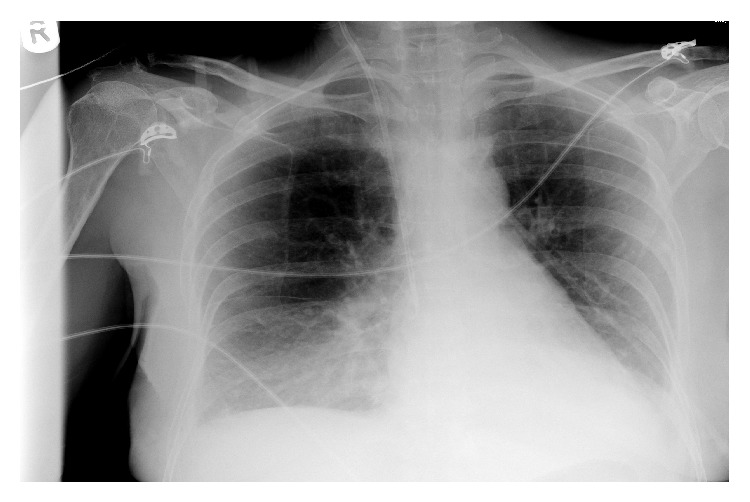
Chest radiograph taken of patient 2 showing right lower lobe consolidation consistent with pneumonia.
